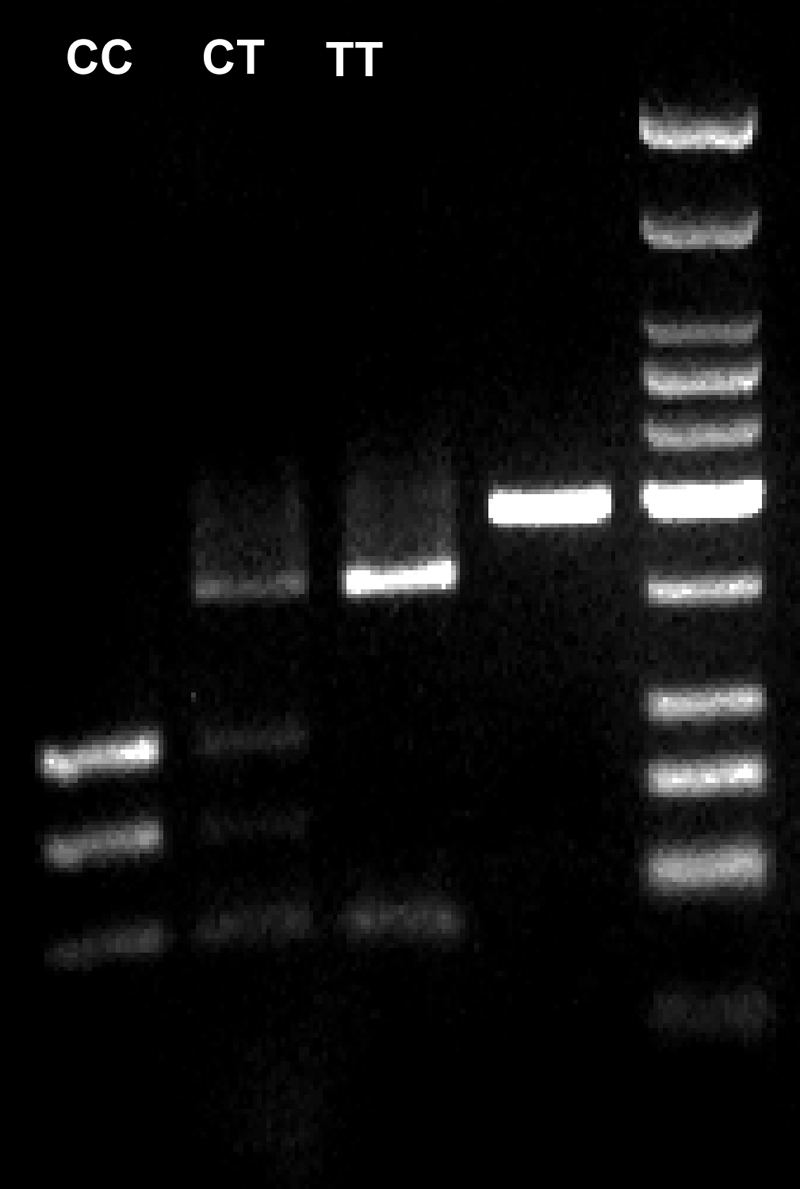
The C1367T polymorphism. Following the use of restriction enzymes, the gel shows: 1, DNA ladder; 2, PCR product well, demonstrating a 195 bp length sequence; 3, TT well, demonstrating two lengths of DNA of 158 bp and 37 bp; 4, CT well, demonstrating 4 DNA lengths of 158 bp, 93 bp, 65 bp, and 37 bp; 5, CC well, demonstrating 3 DNA lengths of 93 bp, 65 bp, and 37 bp (example taken as control).
